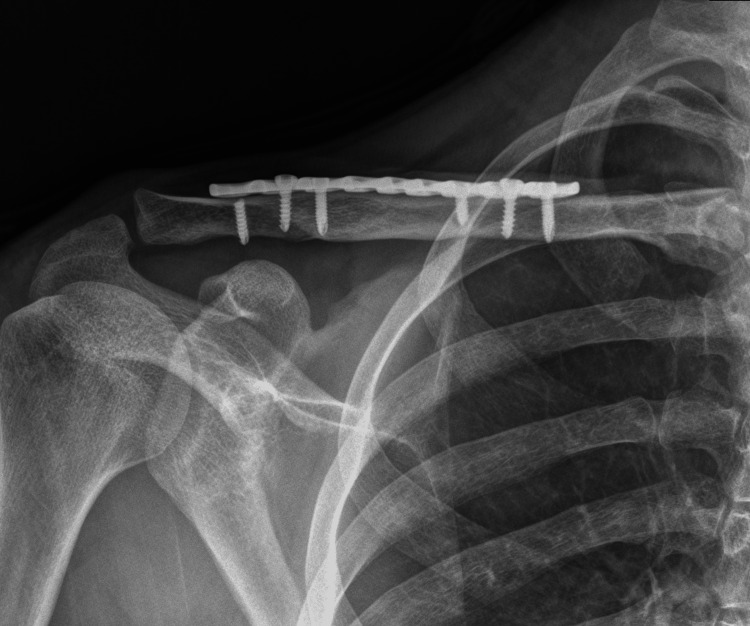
X-ray of the clavicle with the osteosynthesis material.
